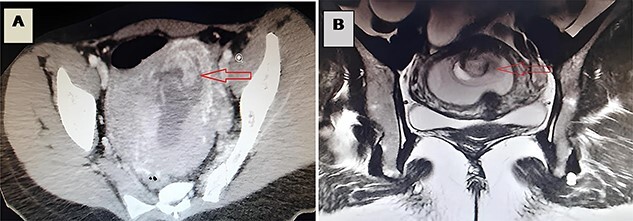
(A) A CT image revealing incarceration of a loop through a defect in the posterior wall of the uterus; (B) an MRI image revealing incarceration of a loop through a defect in the posterior wall of the uterus.
